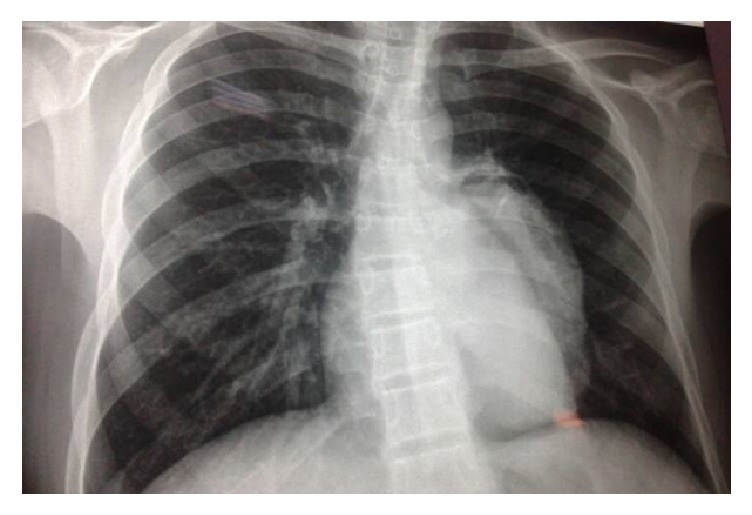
Front view chest X-ray showing a rounded prominence of the left cardiac border with the presence of a radiolucent rim at its superior aspect (crescent sign).
